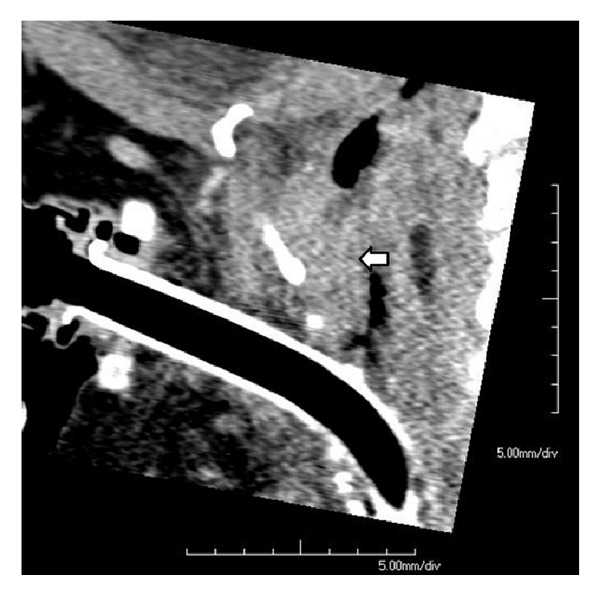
CT scan (sagittal image) of the neck with contrast performed after the initial tracheostomy demonstrates an ovoid mass (arrow) obstructing nearly the entire lumen of the airway. The mass measures 22 mm craniocaudally by 11 mm anteroposteriorly.
